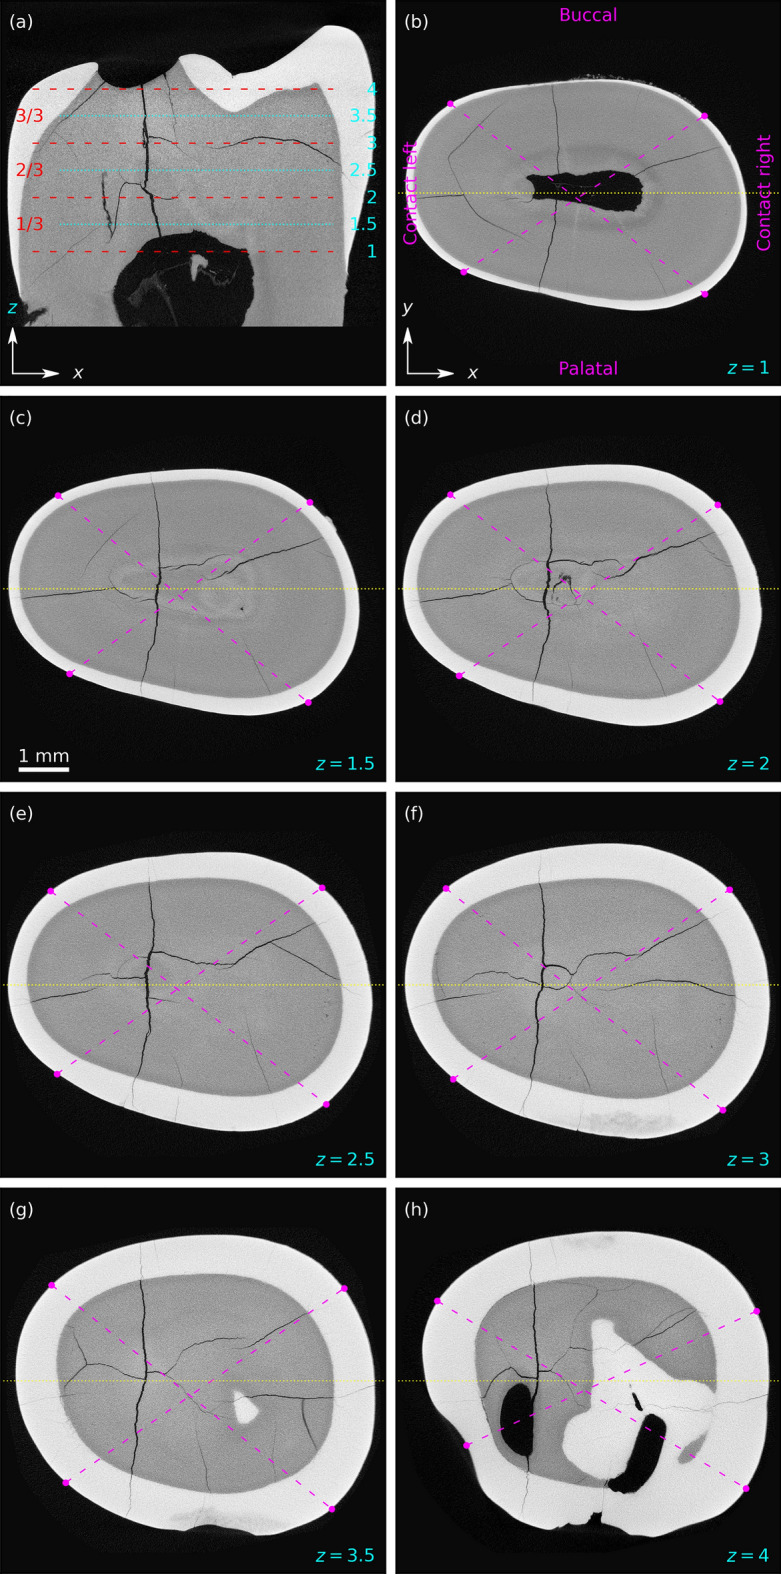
X-ray $$\mu$$CT data-cube cuts of a tooth *without visible* enamel microcracks on the buccal surface. Enamel is light-grey, dentin—dark-grey, and tooth outside, pulp and cracks—black. (a) Vertical slice in *x*, *z* plane at *y* position, as indicated by horizontal dotted yellow line in panels (b–h). (a) Horizontal dashed red and dotted cyan lines show positions $$z=1 - 4$$ of slices in *x*, *y* planes, displayed as images in panels (b–h). (a) Horizontal slabs selected between dashed red lines are indicated as 1/3, 2/3, 3/3. In panels (b–h) dashed magenta lines cut-out four identified sectors (buccal, contact right, palatal, contact left). Field of view is $$\sim 8\times 8$$ mm$$^2$$. One pixel corresponds to $$\sim$$10 $${\upmu \hbox {m}}$$.
